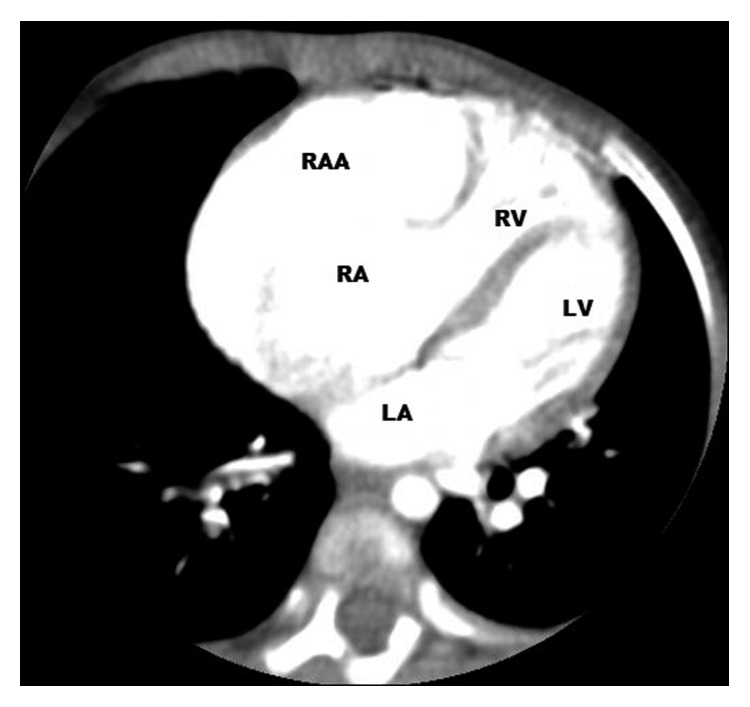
Right atrial aneurysm by CT angiography. LA: left atrium; LV: left ventricle; RA: right atrium; RAA: right atrial aneurysm; RV: right ventricle.
